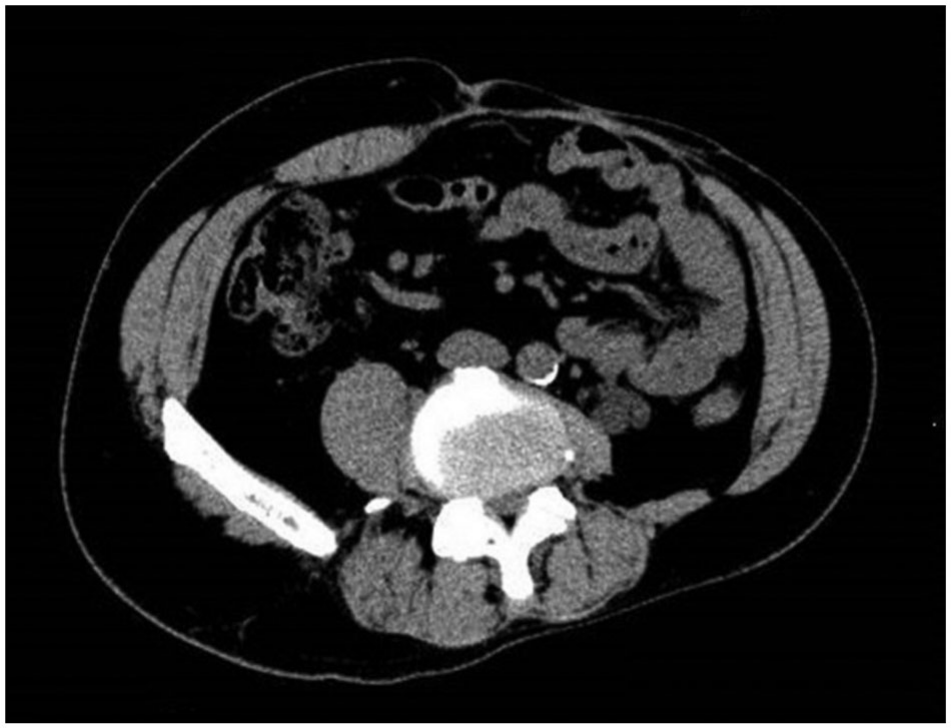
Axial CT image of the abdomen 13 months after last surgery. There is no sign of recurrence of the hernia.
